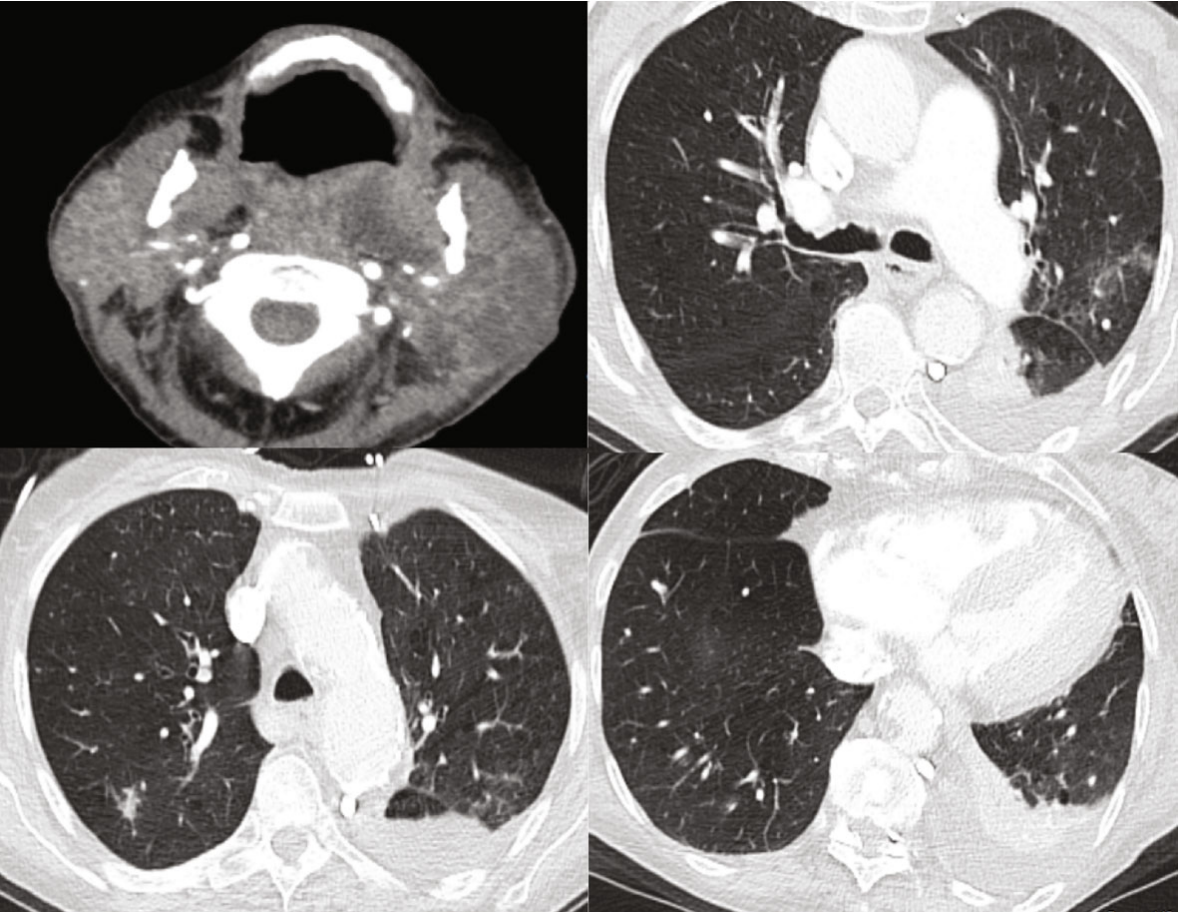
Computed tomography images of case #4.
